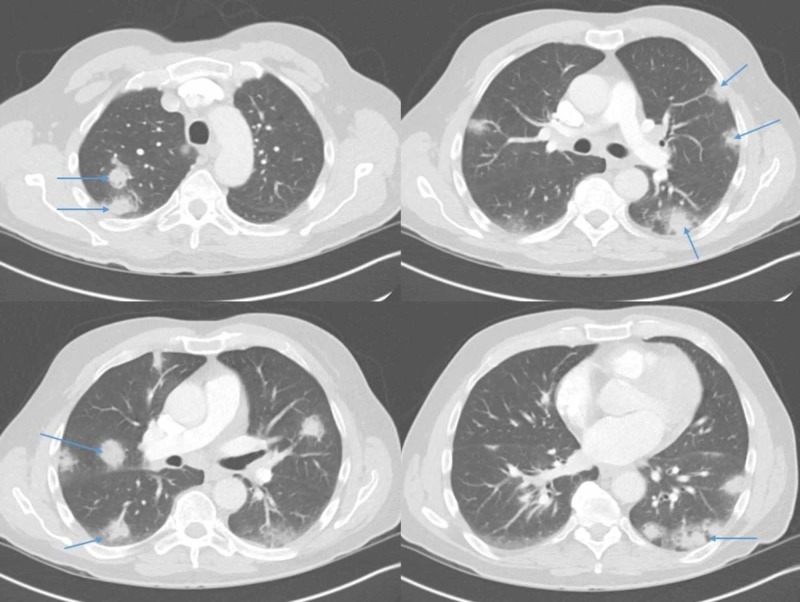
CT scan of the chest revealing numerous lung nodules seen in both lungs at multiple levels as demonstrated in the figure panes (see arrowheads).
